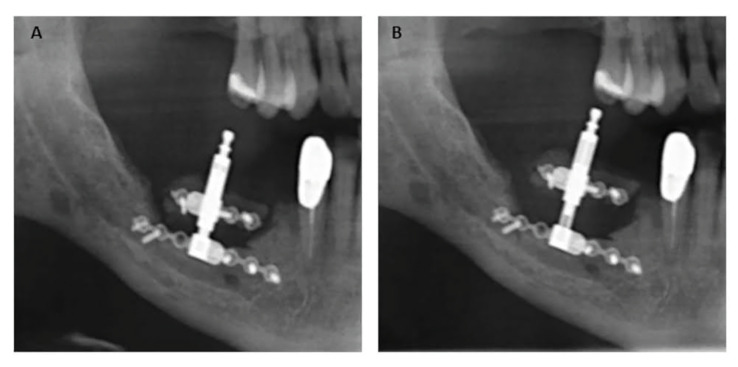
A) Panoramic radiograph taken before the beginning of the distraction period B) Panoramic radiograph taken at the end of the consolidation period. The magnification factor was determined by comparing the size of rods in both panoramic radiographs.
